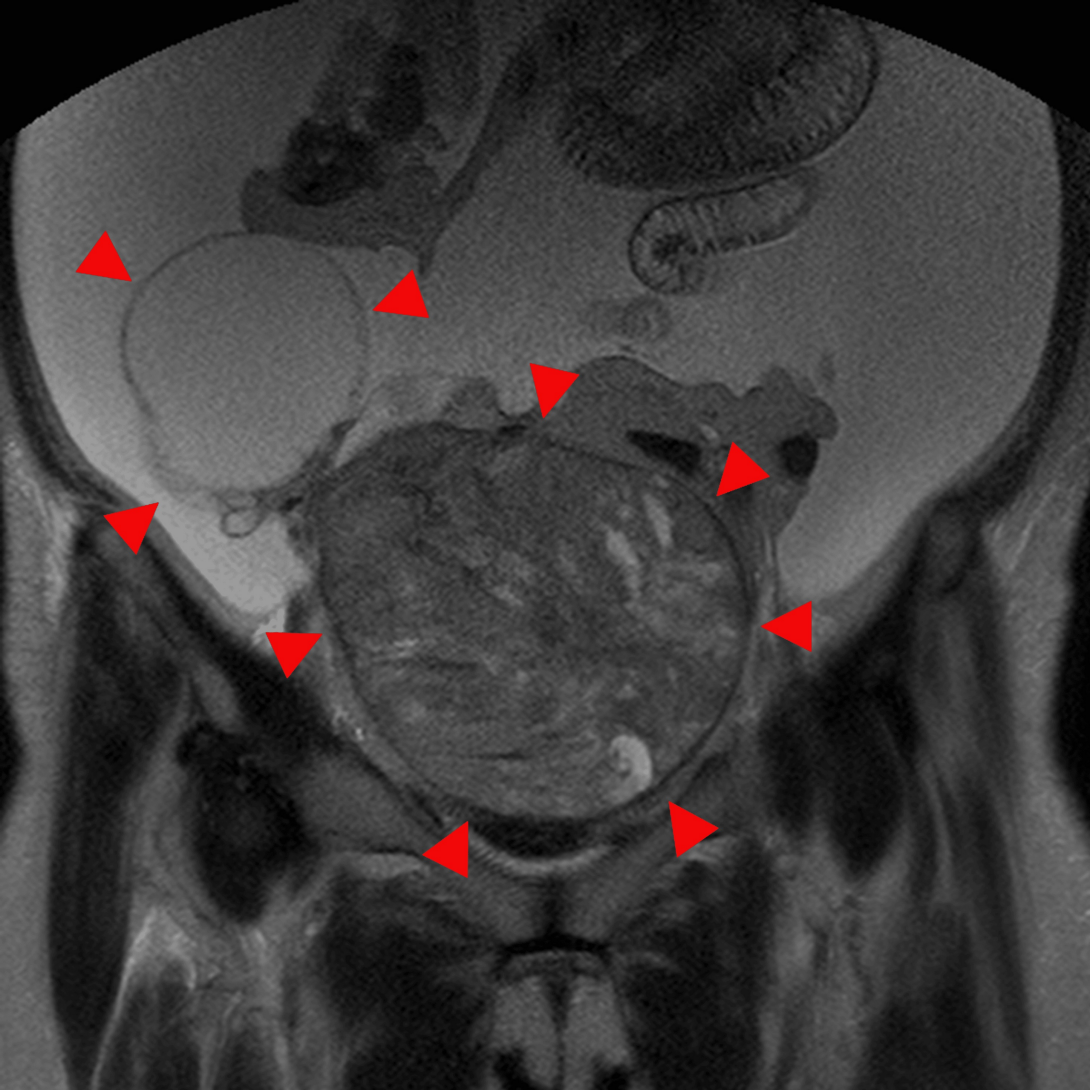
MRI in a 66-year-old woman with ovarian carcinosarcoma of the right ovary The coronal T2-weighted image shows a mass containing both solid and cystic components, accompanied by ascites (arrowhead). The tumor measures 21.5 cm in diameter.
